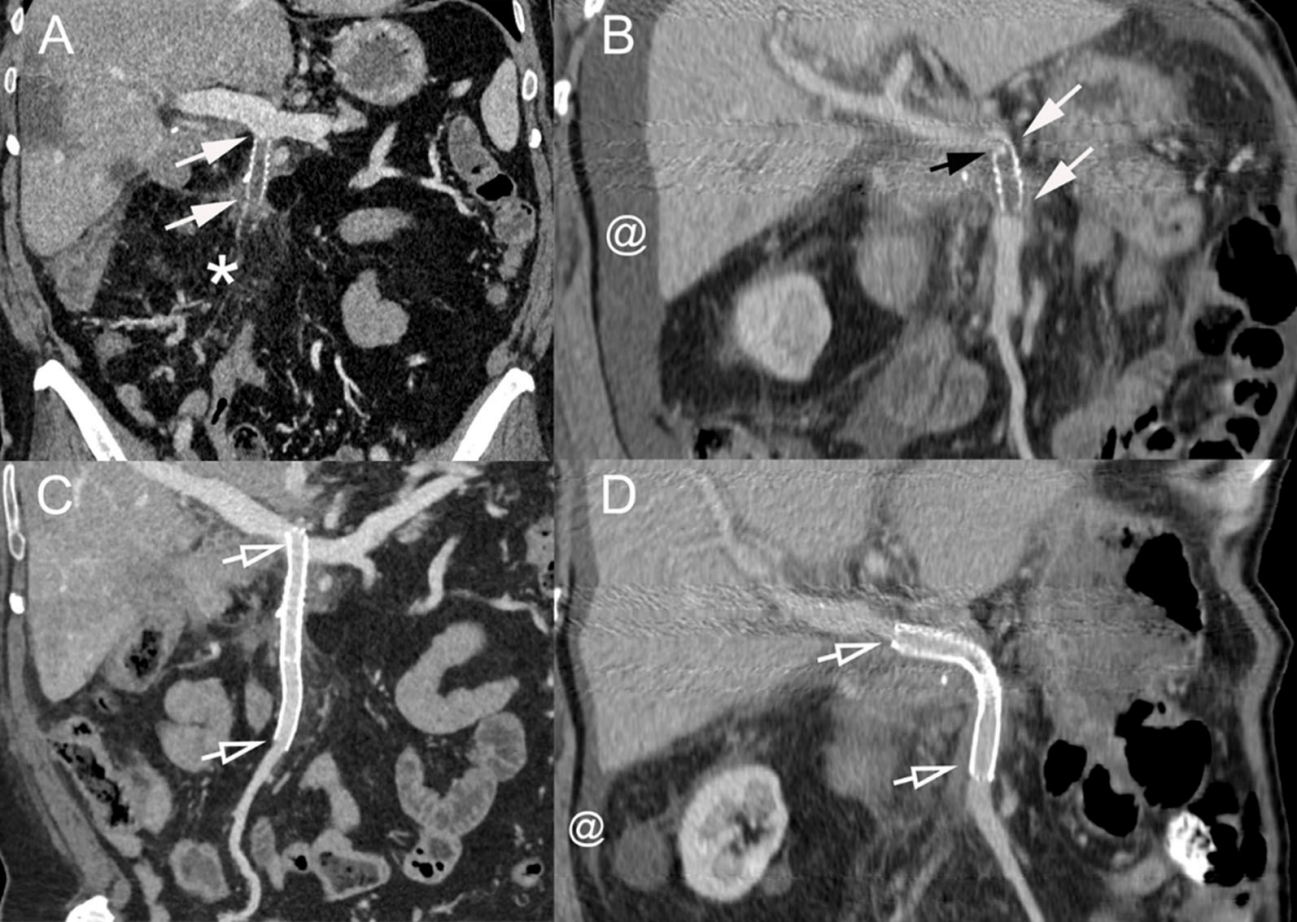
(A) Coronal CT image (portal venous phase) of a 60-year-old man with pancreatic cancer treated with PD with distal SMV resection and prosthetic graft placement presenting with asthenia, weight loss, and abdominal pain. The prosthetic graft contains hypoattenuating material consistent with thrombosis (arrows). Fat stranding is also present around the mesenteric vessels and graft (*). (B) Coronal CT scan image (portal venous phase) in a 75-year-old man with pancreatic cancer treated with PD with distal SMV resection and prosthetic graft placement presenting with asthenia and abdominal distention due to ascites. The graft is thrombosed (arrows) due to a stenosis at the cranial anastomosis (black arrow). Ascites is present (@). (C, D) Coronal CT scan image (portal venous phase) after percutaneous transhepatic recanalization and stenting of the occluded grafts of patients [(A, B), respectively]. Stents are patent (hollow arrows) with disappearance of the fat stranding in panel (A) and marked decrease of the ascites in panel (B) (@). PD, pancreatoduodenectomy; SMV, superior mesenteric vein.
